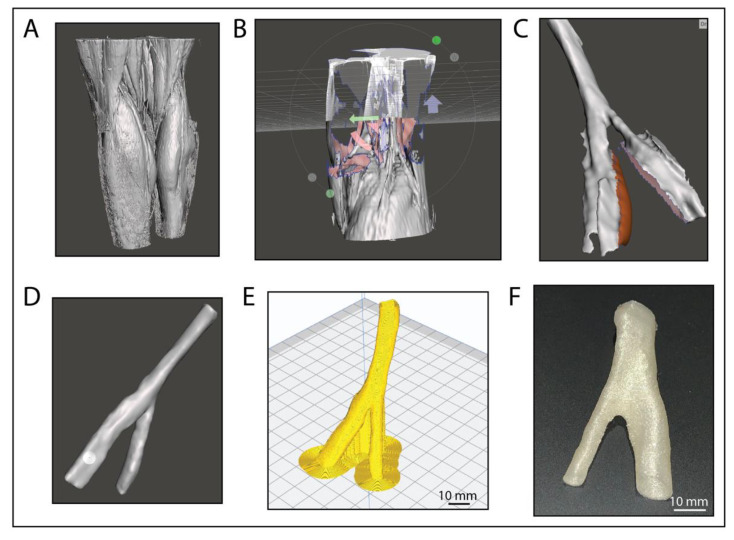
(A) Muscle and nervous tissue isolated in CT scan and converted to a 3D model; (B) Isolating sciatic nerve from surrounding soft tissue using selection and deletion tools; (C) Further isolation of the sciatic nerve and the removal of excess tissue; (D) Smoothing of sciatic nerve using sculpting tools and hollowing using the make hollow tool; (E) Slicing nerve conduit in slicer for 3D printing. (F) Photograph of the 3D Printed sciatic nerve conduit 0.2 mm nozzle diameter printed at 215 °C.
